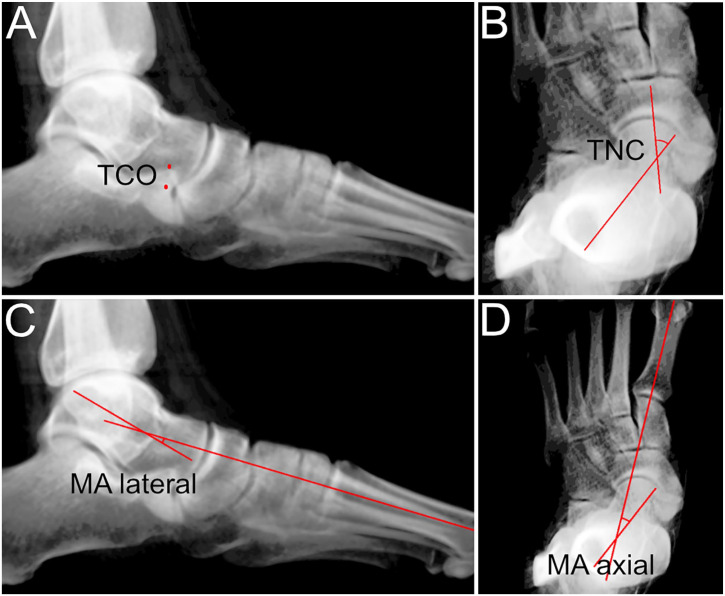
Semiautomated 3D measurement based on weightbearing computed tomography scans. (A) Lateral image of the foot showing the overlap between the calcaneal anterior process and the talus (TCO). (B) Dorsoplantar (DP) image of the foot showing talonavicular coverage (TNC) angle. (C) Lateral foot image showing lateral Meary angle (MA lateral). (D) DP image of the foot showing axial Meary angle (MA axial).
